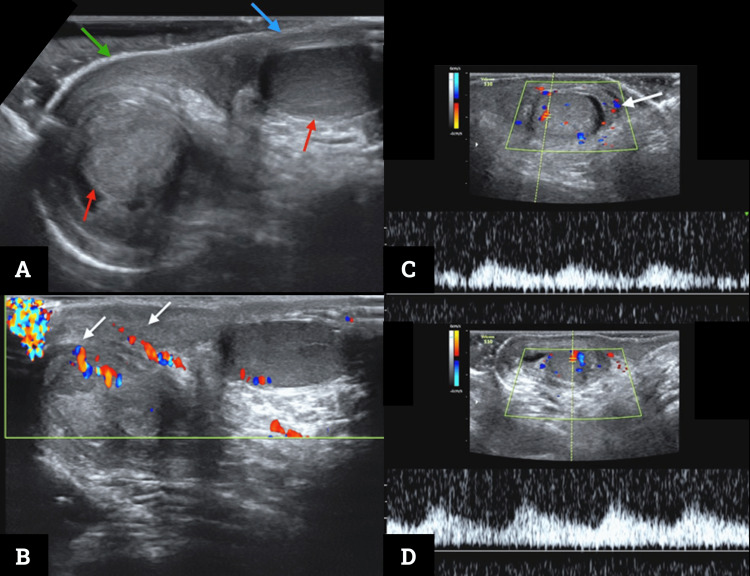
Testicular Doppler ultrasound (A) B-mode examination transverse plane in the scrotum with both testes in the scrotal sacs (red arrows). Asymmetrical thickening of the scrotum on the right side (green arrow) compared to its contralateral (blue arrow). (B) Color Doppler ultrasound oblique plane through the scrotum. Increased color Doppler signal in the right scrotum (white arrows) secondary to hyperemia and configuring the “fountain sign.” (C) and (D) Color and spectral Doppler ultrasound in the longitudinal plane to the right and left testis, respectively. Spectral analysis shows symmetrical and normal low resistance waves, typical of standard testicular circulation—the increased color Doppler signal from the right scrotum is persistent (white arrow in panel C).
